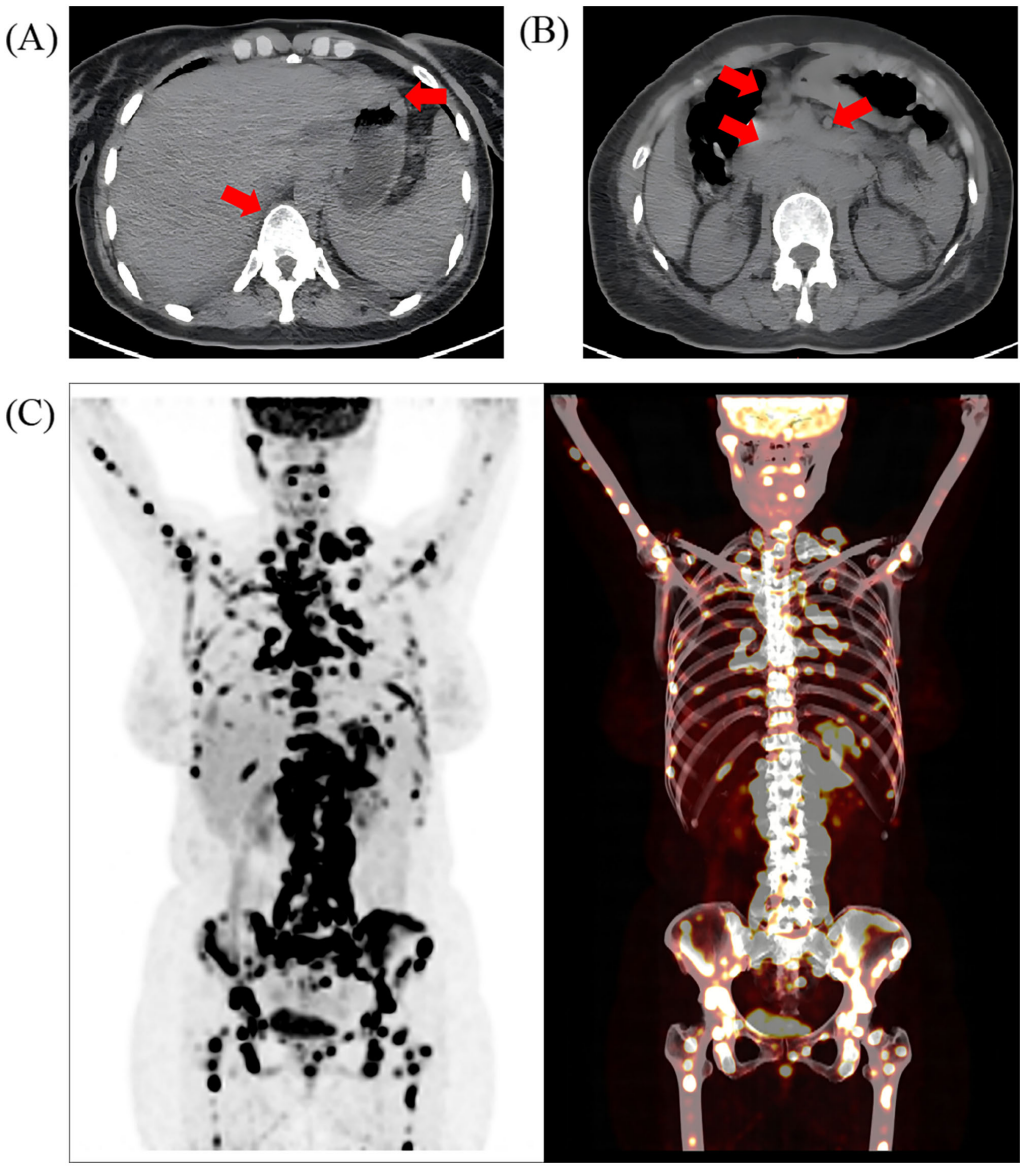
The imaging evaluation of the patient at admission. Positron emission computed tomography (PET-CT) showed (A) thickening of gastric wall as well as mixed metastasis of the vertebras and (B) multiple enlarged abdominal lymph nodes; the red arrow represents the location of the lesion; (C) increased FDG uptake in multiple areas of the bone marrow, including the skull, right mandible, several vertebrae and their attachments, sternum, multiple ribs, pelvic bones, clavicles, scapulae, humeri, and femurs.
